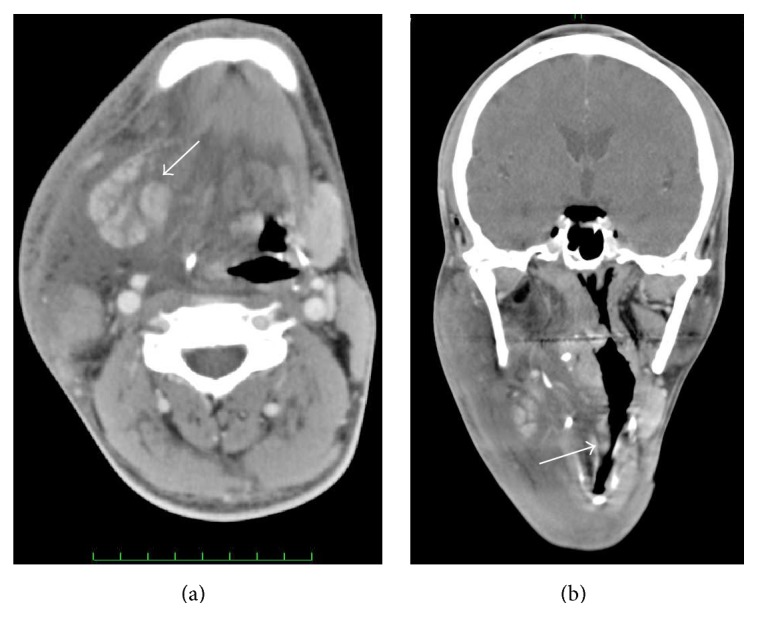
(a) Axial image of computed tomography 7 hours after operation. Edematous tissue around the right submandibular gland (arrow) was found to have increased, and it extended to the right parotid region. (b) Coronal image of computed tomography 7 hours after operation. The glandular swelling was found to be displacing the larynx (arrow).
